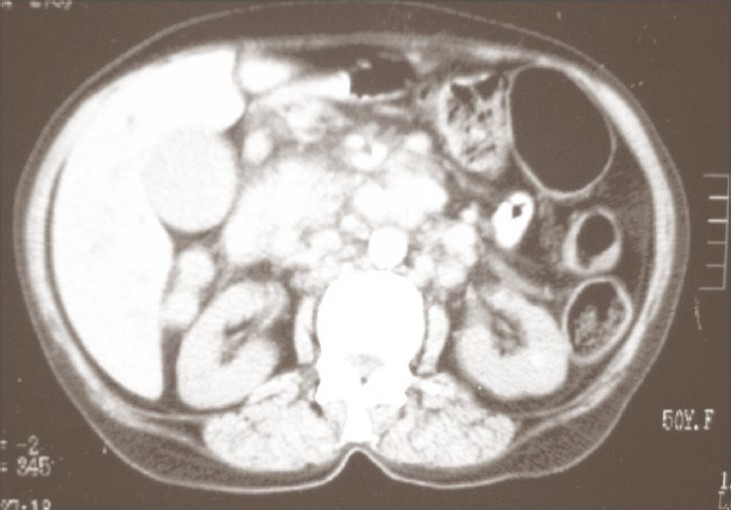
CT scan showing enlarged head of pancreas with heterogeneous soft tissue mass measuring 5 × 5 cm. with multiple porta hepatis and paraaortic lymph nodes with no evidence of hepatic focal lesions
